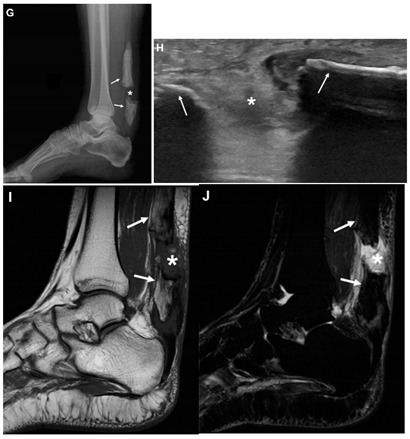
Sullivan and Thurston, 2021 ^e^ [35]	A 72-year-old man. This image submission includes an ultrasound (Image K), lateral view x-ray (Image L), and an MRI (Image M) of a patient who presented to an orthopedic sports medicine clinic after feeling a “pop” while mowing his lawn. Image K is an ultrasound of the Achilles Tendon that depicts a segment of discontinuity (green arrow) within a linear hyperechoic signal. Image L and Image M show ossification of the Achilles Tendon and arrows (red and blue, respectively) indicate fracture of the ossified mass. The patient had no knowledge of the ossification, but he reported having lower leg surgery as a 2-year-old to correct for in-toeing. The patient was treated conservatively with casting and physical therapy. One year after the ossification and coexisting fracture of the ossified mass was discovered, he reported being fully functional without pain.
